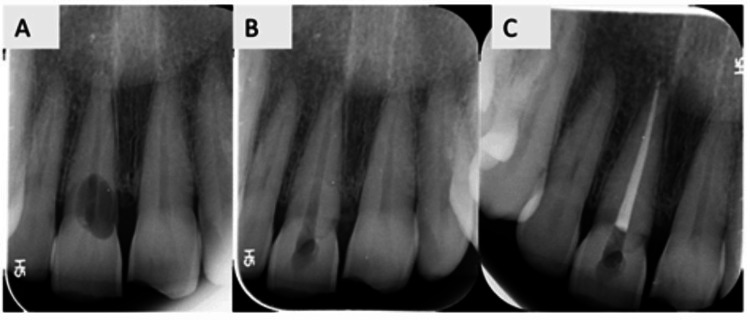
(A) Preoperative IOPAR, (B) postoperative radiograph after one week of surgical repair, and (C) IOPAR after root canal obturation IOPAR: intraoral periapical radiograph
